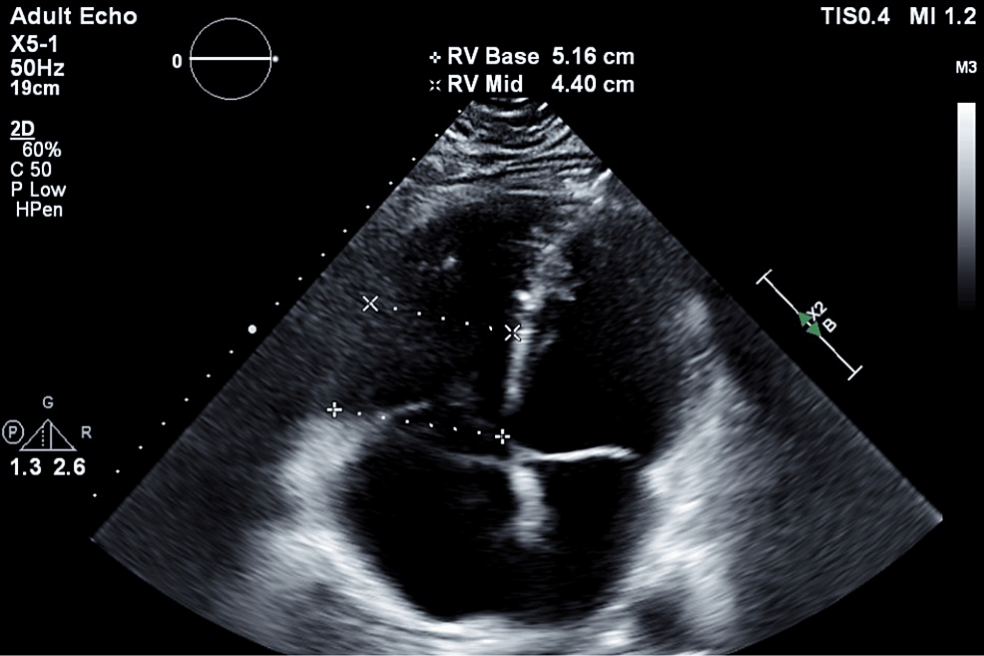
The Echo reveals an enlarged RV and right atrium, along with a flattened interventricular septum (from last admission in 2024) Echo: Echocardiogram; RV: Right ventricle
